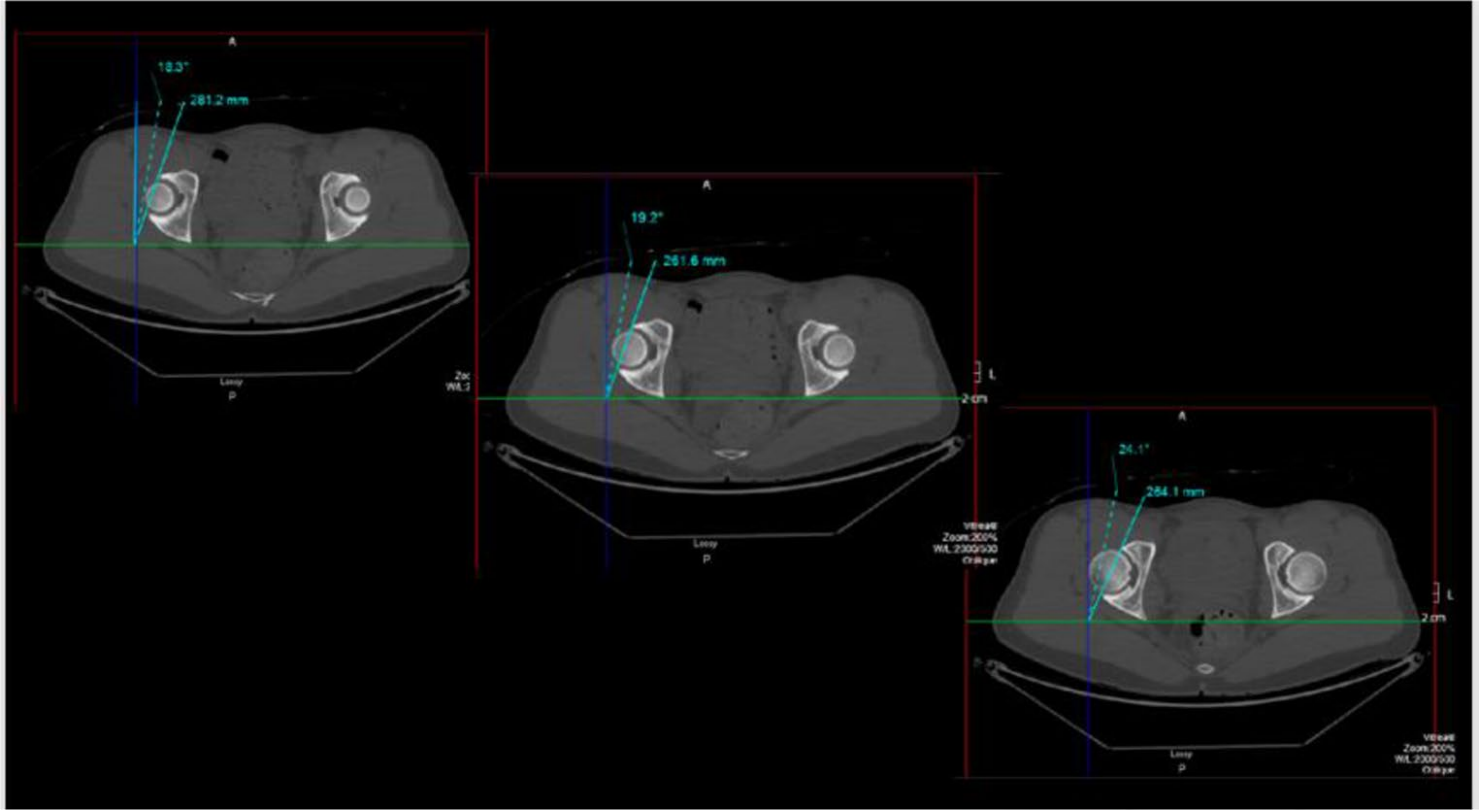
Acetabular version at the 1, 2 and 3 o’clock position measured off the axial CT slices of the acetabulum
